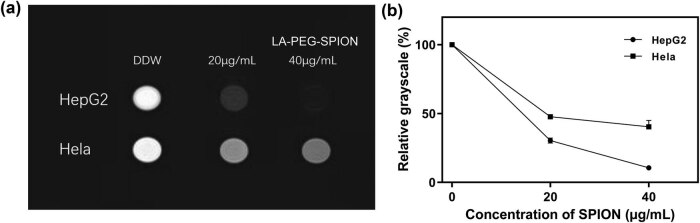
In vitro* MRI. (a) T2WI images of *in vitro* MRI. (b) Quantification of the MRI signal by gray value. DDW, deuterium depleted water.
